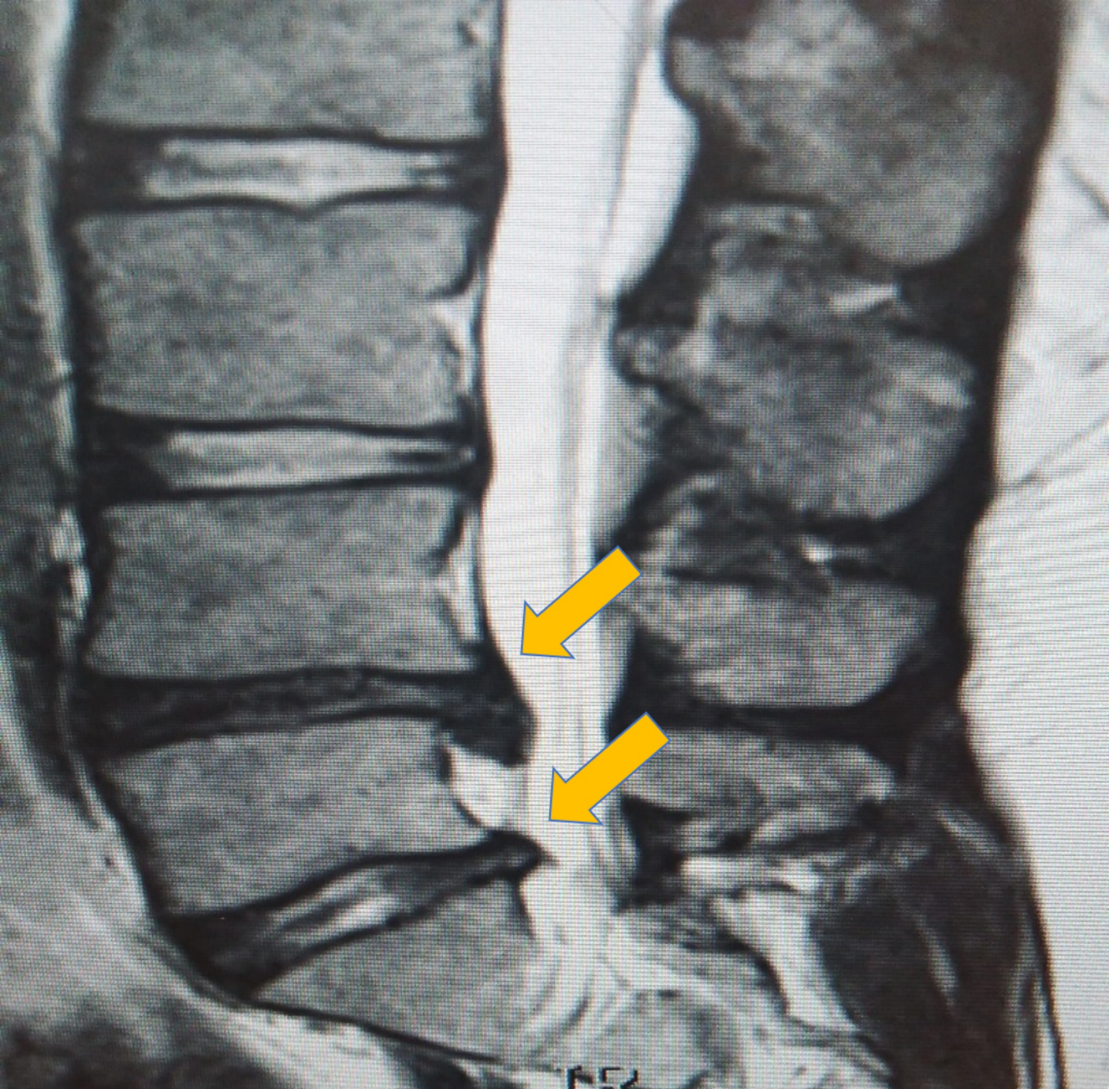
Magnetic resonance image of disc prolapse (sagittal view) Large L4-L5, L5-S1 disc bulges
